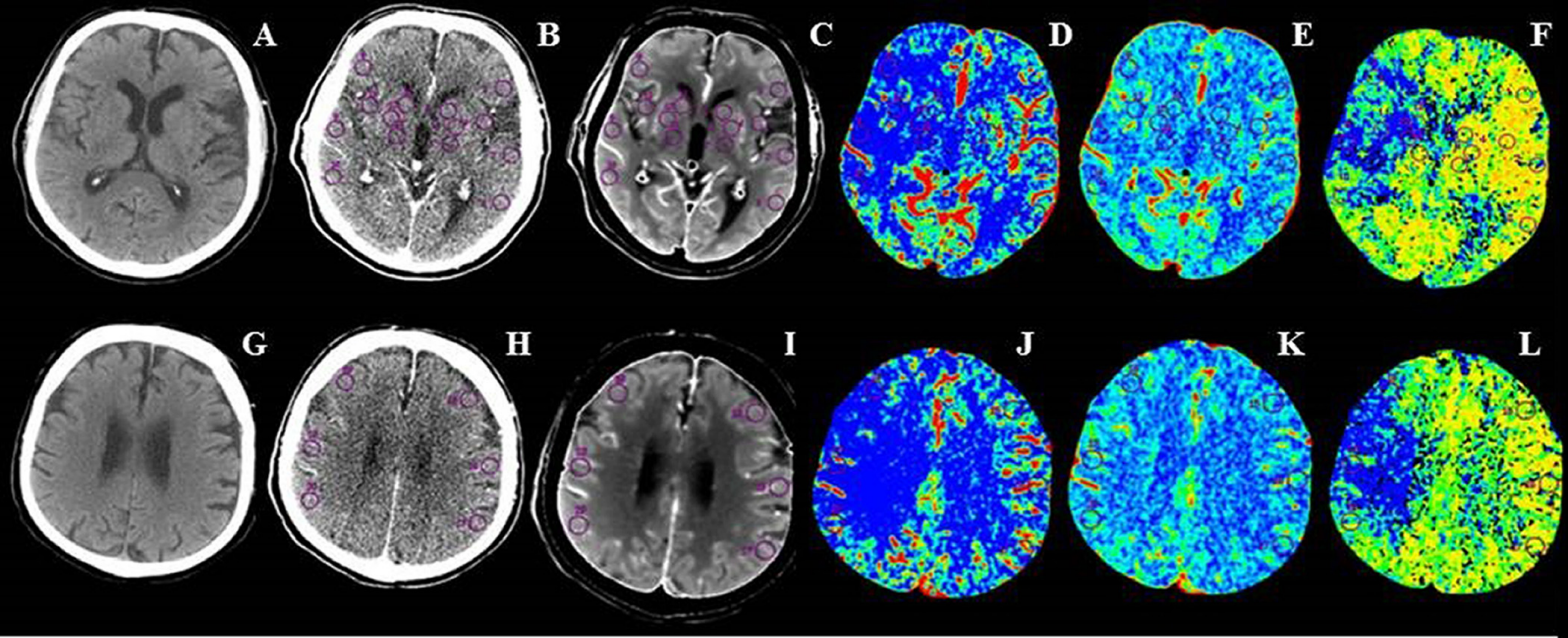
Non-constrast CT, baseline source and average CT perfusion images, cerebral blood flow (CBF), cerebral blood volume (CBV) and mean transit time (MTT) maps at ganglionic (A-F) and supraganglionic (G-L) axial ASPECTS levels. Multiple circular regions of interest (ROIs) larger than 1 cm^2^ placed freehand in the affected hemisphere and automatically reflected into homologous regions of the contralateral hemisphere were used to measure CBF, CBV and MTT absolute values from the corresponding functional maps in ASPECTS regions at ganglionic (anterior middle cerebral artery cortex, middle cerebral artery cortex lateral to insular ribbon, posterior middle cerebral artery cortex, insula, caudate nucleus, lentiform nucleus and internal capsule) and supraganglionic (anterior, lateral, and posterior middle cerebral artery cortical territories immediately superior to the previous ones, rostral to basal ganglia) sections.
